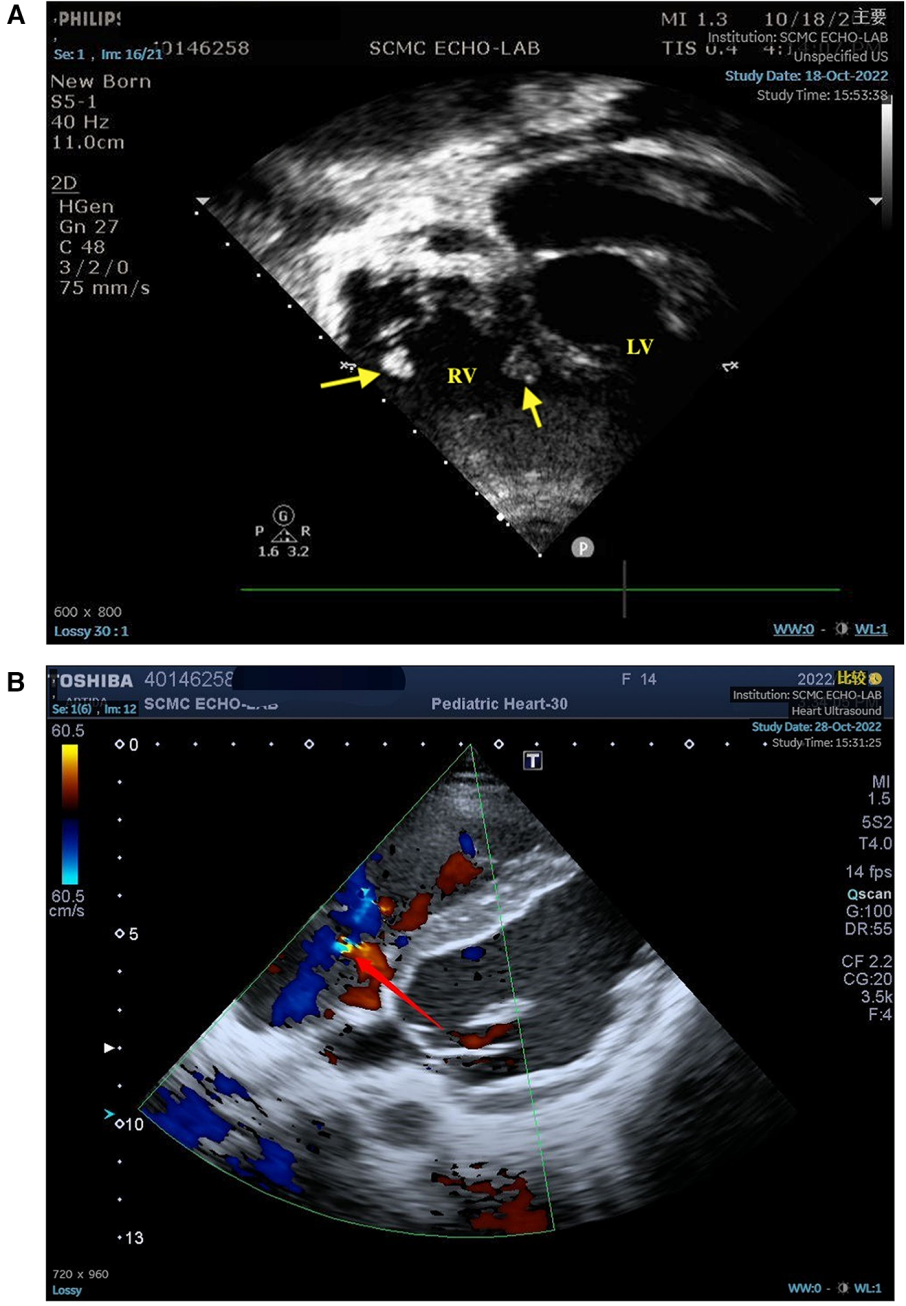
(A) Two moderately echogenic masses attached at the level of the tricuspid chordae tendineae measured in atypical apical four-chamber (4C) view. (B) The yellow part of the arrow indicates turbulence, indicating tricuspid regurgitation. LV, left ventricle; RV, right ventricle; arrow, masses.
